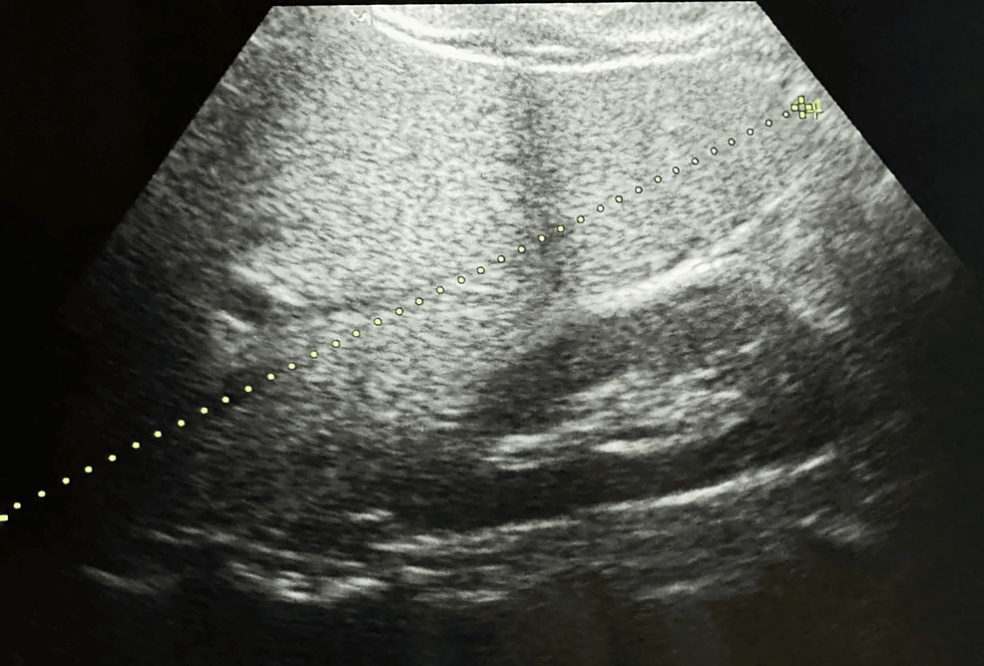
Ultrasonography indicating slightly higher echogenicity than the renal cortex, suggesting grade 1 fatty liver
